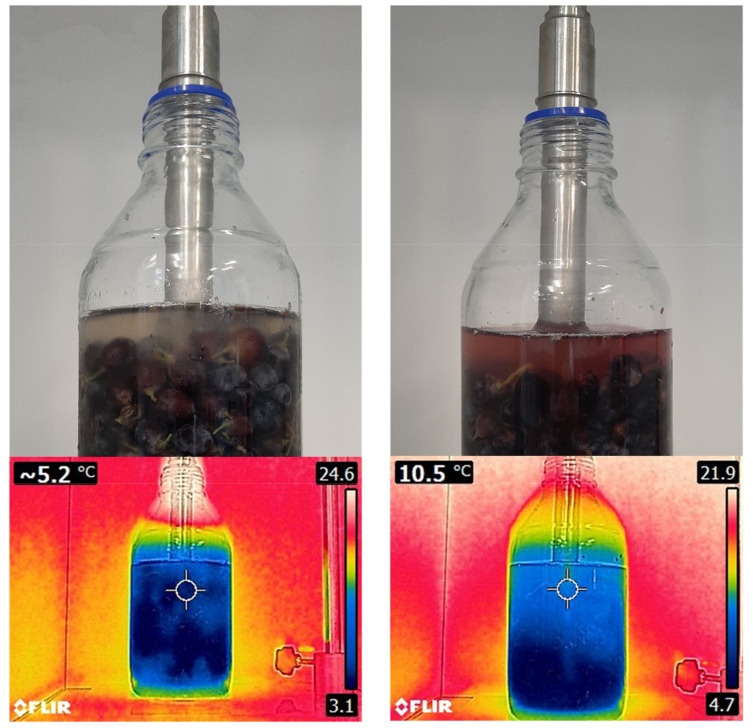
Use of USs in the extraction of grape anthocyanins and effect on temperature measured with an infrared camera. Left: before ultrasonication, right: after US treatment.
